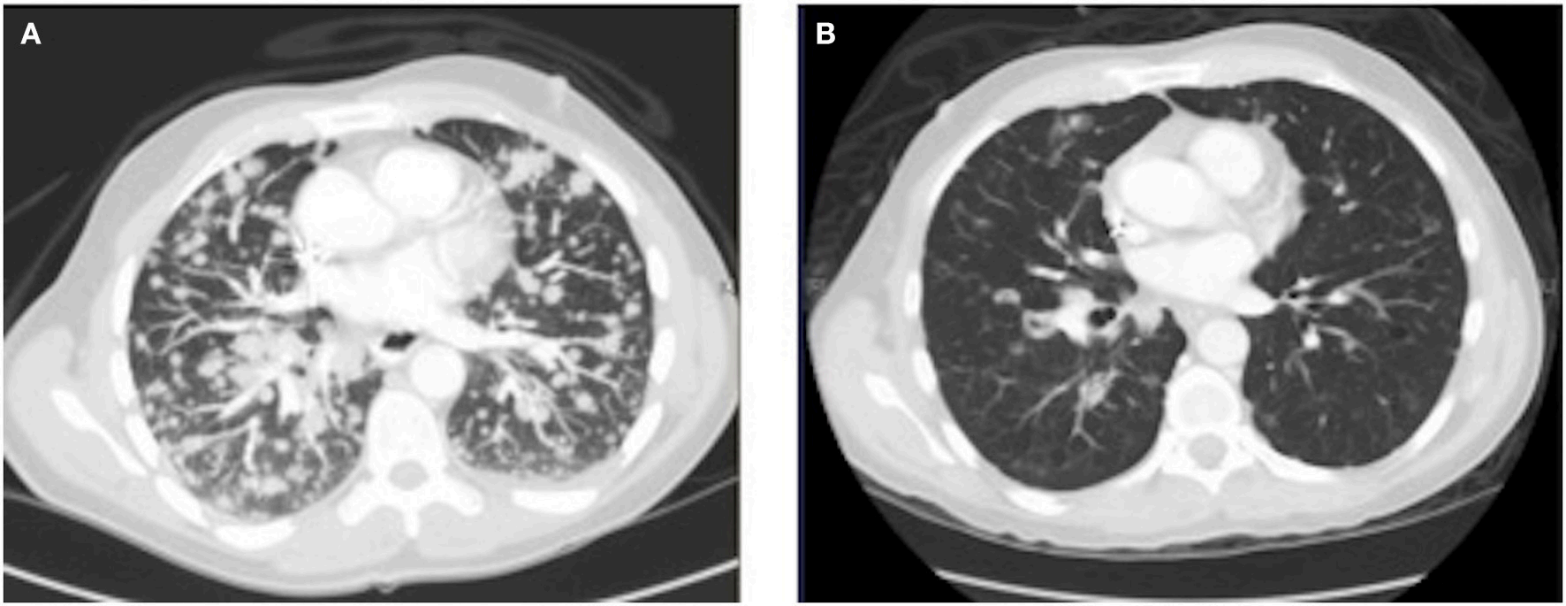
Chest CT image (A) before the start of cabozantinib plus panitumumab and (B) after 42 days of cabozantinib plus panitumumab.
